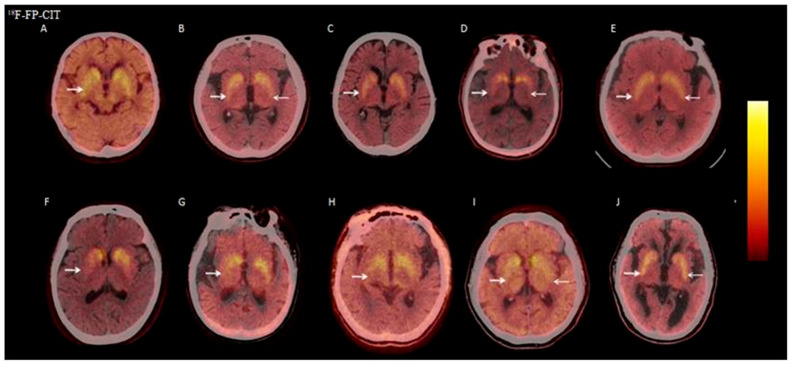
Eleven DLB patients underwent ^18^F-FP-CIT PET/CT, with 10 (10/11, 90.9%) showing reduced basal ganglia dopamine transporter distribution. (A,C,F–H) show asymmetric bilateral reduction, more severe on the right. (B,D,E,I,J) show symmetric bilateral reduction.
